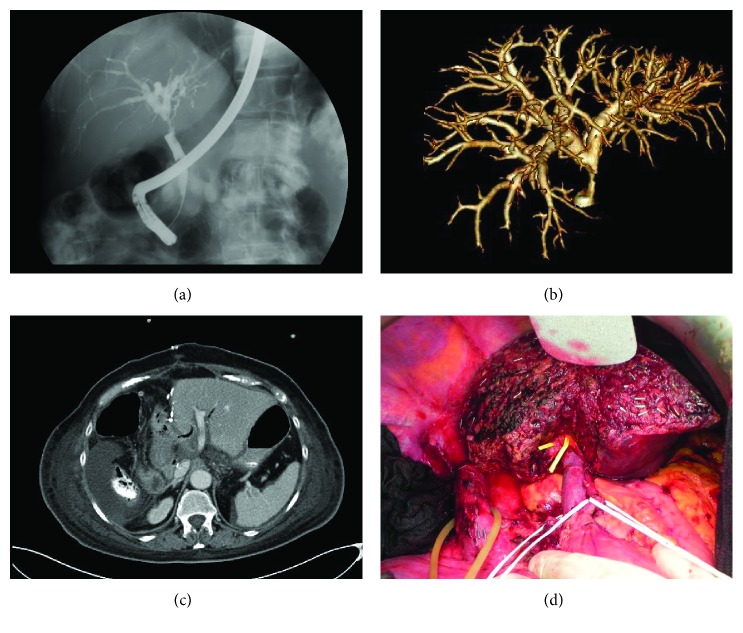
Preoperative imaging and intra- and postoperative status after hepatectomy. (a) Conventional ERC, patient with Klatskin tumor bismuth type II. (b) 3D-ERC, patient with Klatskin tumor bismuth type II. (c) Axial CT scan 4 days after extended right hepatectomy. (d) Intraoperative status after right trisectionectomy.
